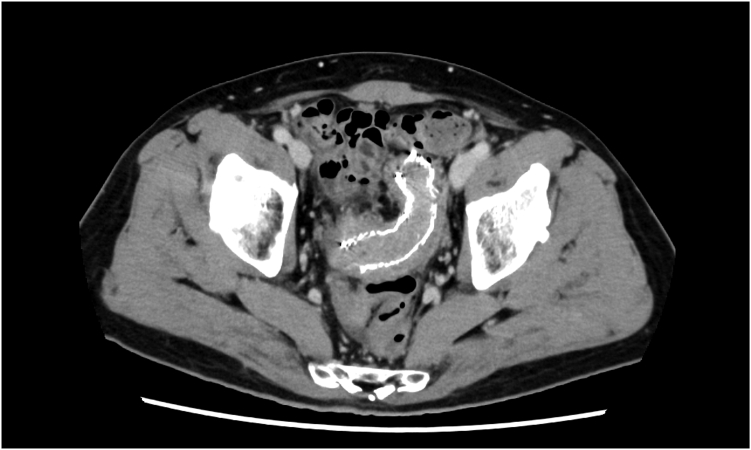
A CT scan revealed a colonic stent occlusion due to tumor ingrowth.
